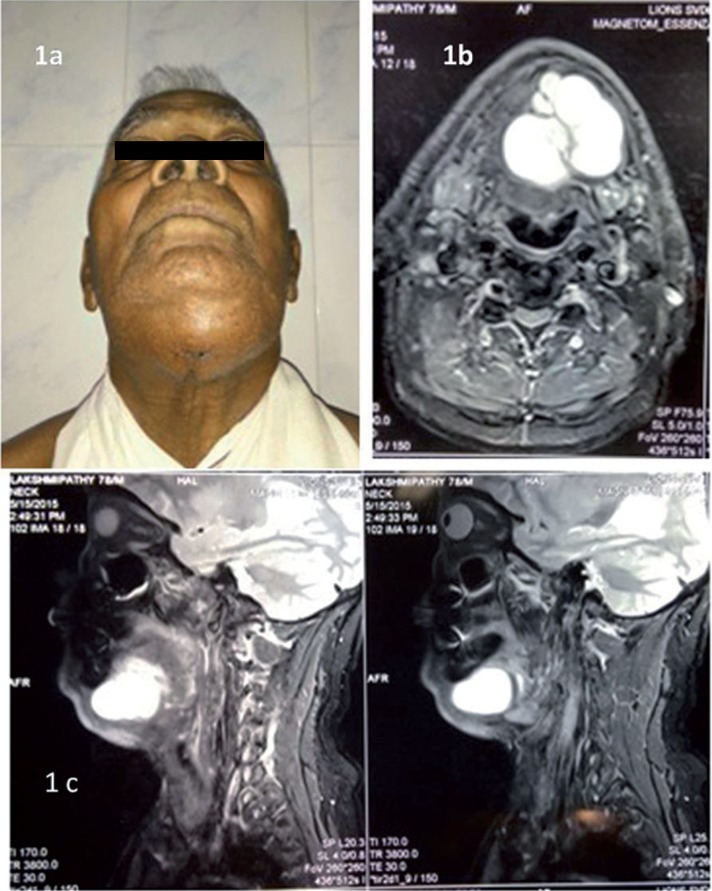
a) Extraoral view showing swelling in the submental region extending bilaterally to the submandibular region. b,c) Magnetic resonance imaging axial and sagittal view showing well defined altered signal intensity soft tissue mass lesion involving the floor of the mouth with extension into the submental region displacing the left submandibular gland.
